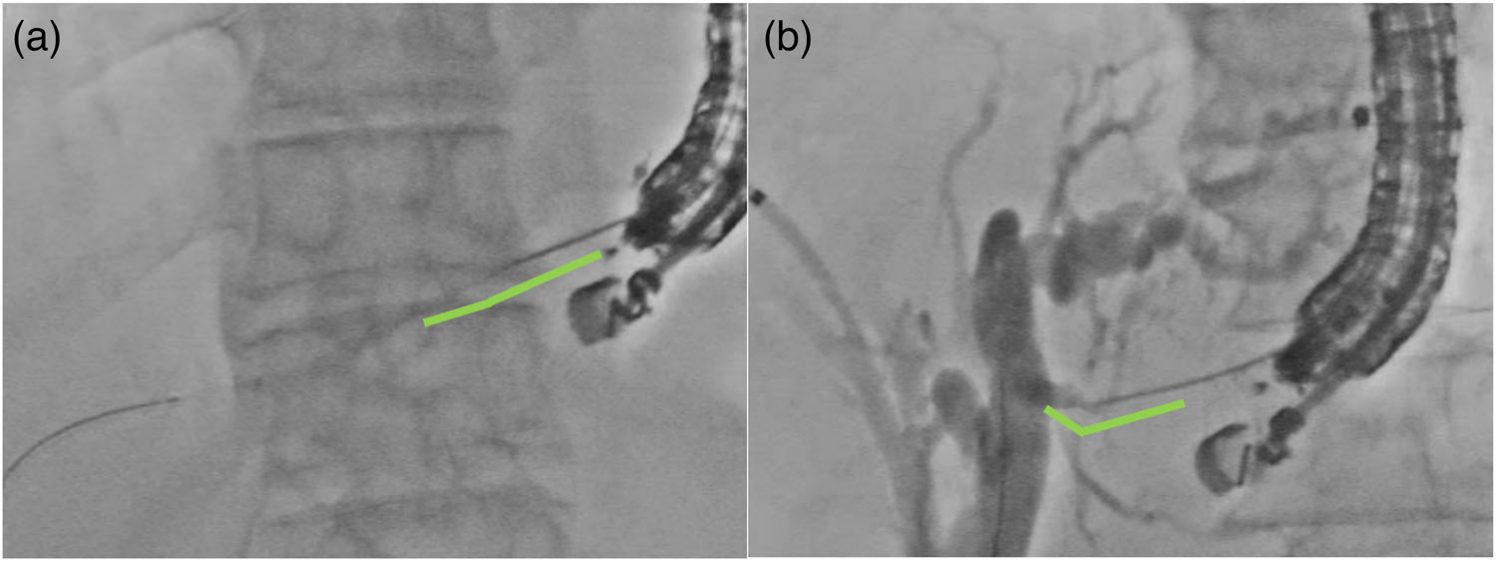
The comparison of the puncture angle for both B2 (a) and B3 (b) in the radiographic image
